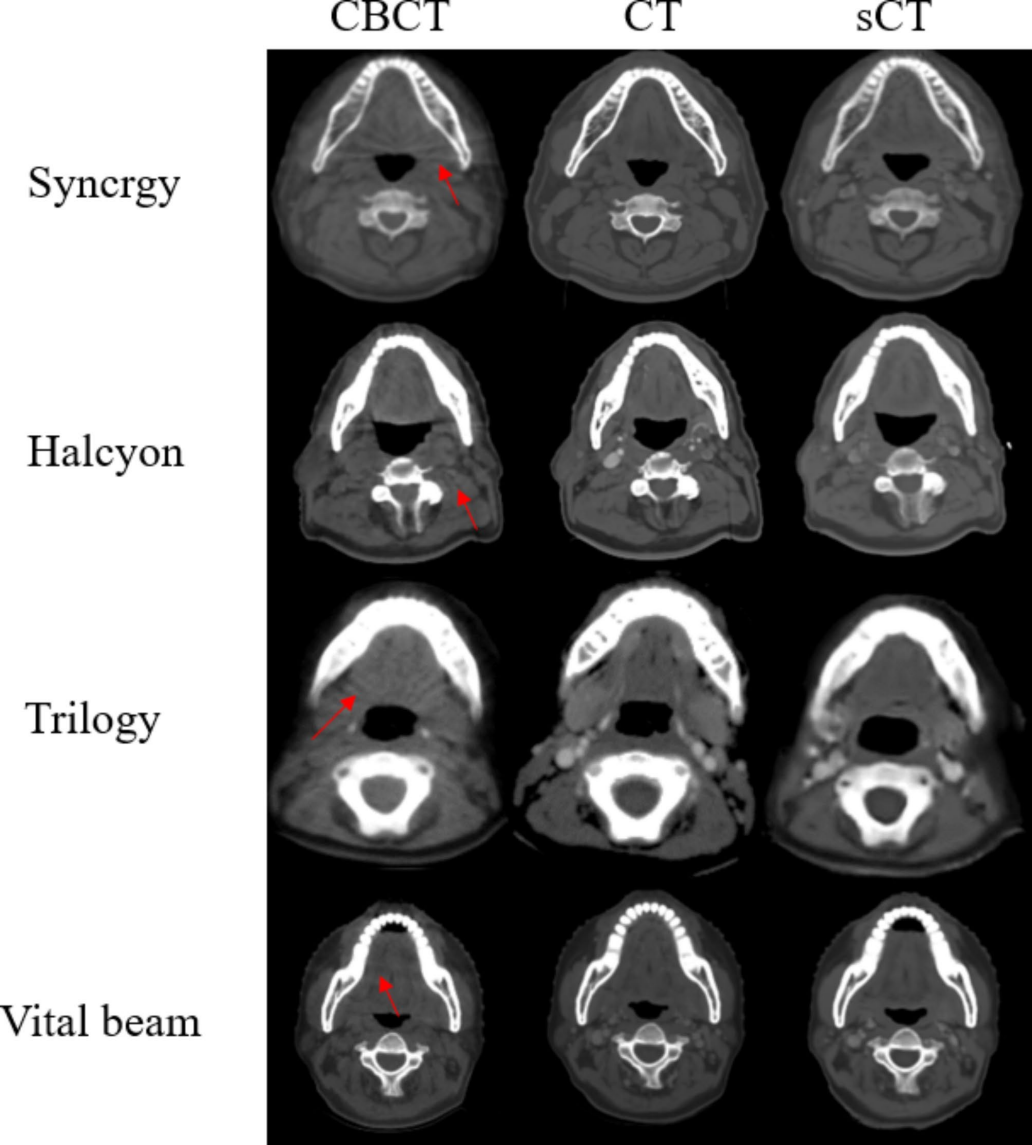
Comparison among the CBCT, CT, and sCT images for different accelerators
